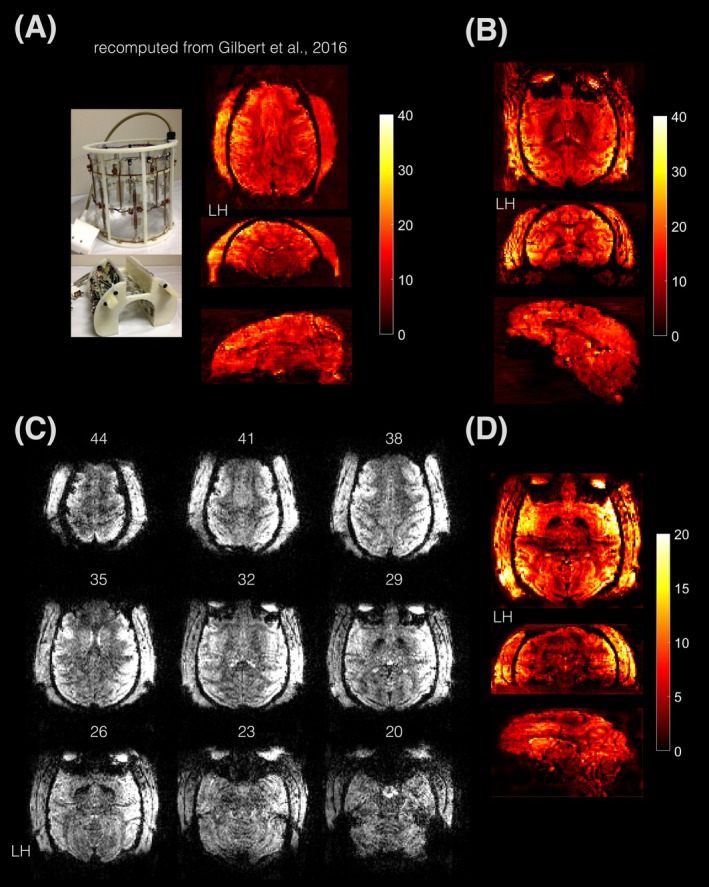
tSNR comparison at 1 mm resolution and functional MRI image quality from the hybrid coil at submillimeter resolution. (A) tSNR map computed from 600 TR acquisition (TR/TE = 1000/18 ms, *R* = 2 × 2, PF = 3/4, BW = 1680 Hz/px) at 1 mm isotropic resolution adapted from Gilbert et al. [17] accessible on the PRIME‐DE database. (B) tSNR map derived from our 600 TR EPI acquisition (TR/TE = 1960/24 ms, *R* = 2 × 3) at 1 mm isotropic resolution from the hybrid coil. (C) Raw EPI images from the 2D‐SMS acquisition at 0.75 mm isotropic resolution from the hybrid coil. Slice numbers are indicated on top of each slice. (D) tSNR map derived from 600 TR acquisition at 0.75 mm isotropic resolution across the three planes.
